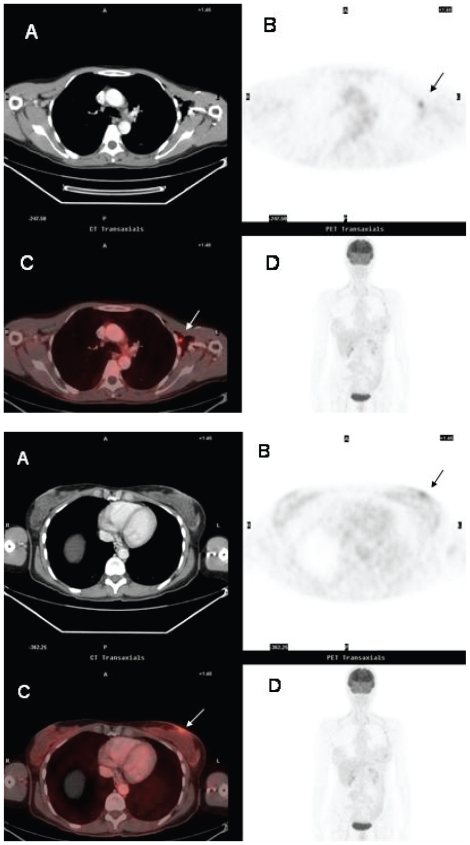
False-positive FDG-uptake in viral infection. Forty-seven year-old woman referred for staging of T-cell non-Hodgkin’s lymphoma. ^18^FDG PET (B, D) ^18^FDG PET/CT fusion (C) scans shows abnormal foci of ^18^FDG uptake in the left axilla (2A) (arrow) and the skin overlying the left breast (2B) (arrow). The findings were misinterpreted as cutaneous and nodal lymphomatous infiltration. Skin and axillary node biopsies demonstrated herpes virus infection and benign reactive follicle hyperplasia respectively. There was no evidence for malignancy.
